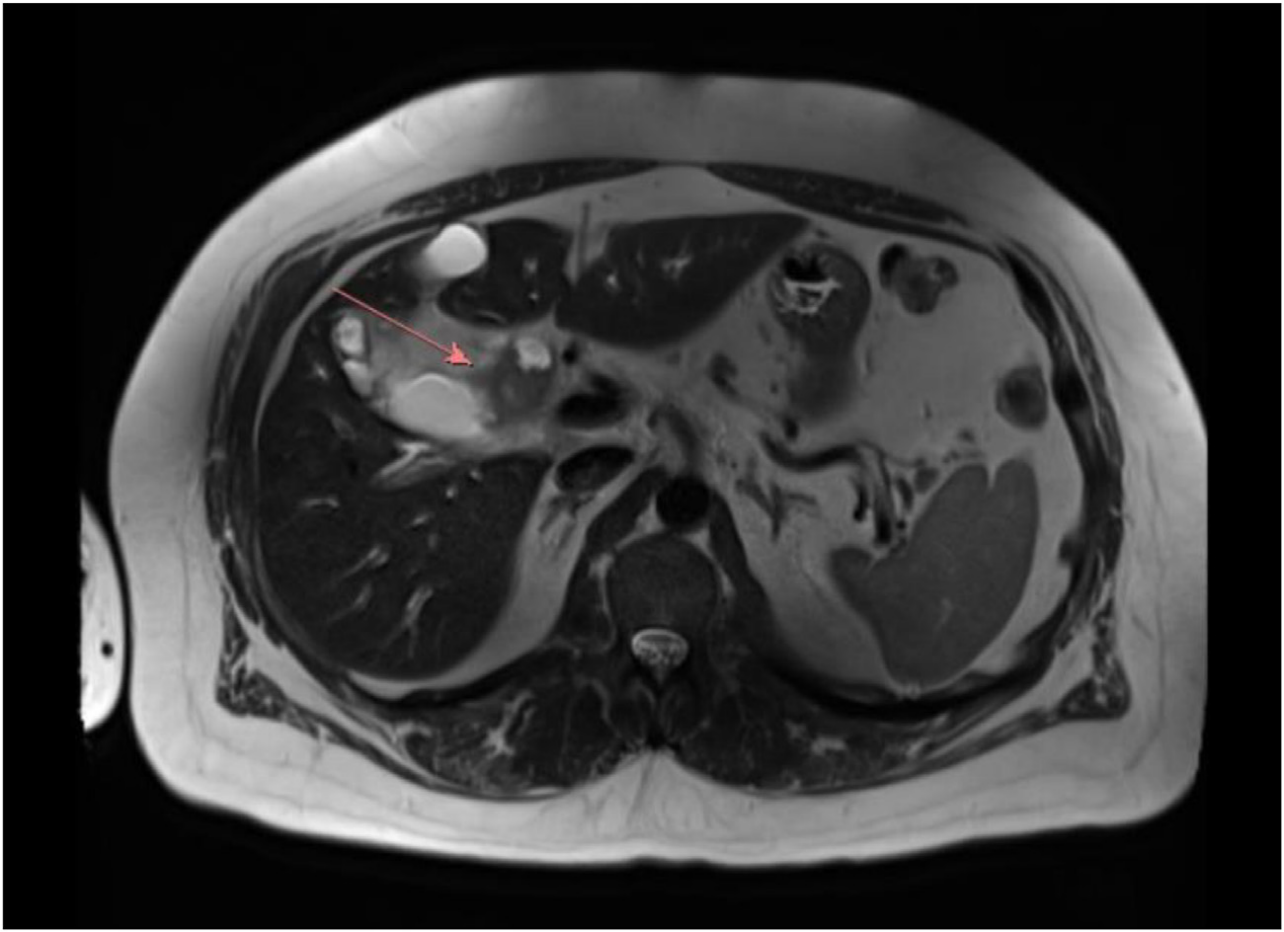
The same T2 weighted MRI/ MRCP with axial image through the upper abdomen again shows focal, mass-like wall thickening of the distal common hepatic duct (red arrow) with upstream dilation of the proximal common hepatic duct.
